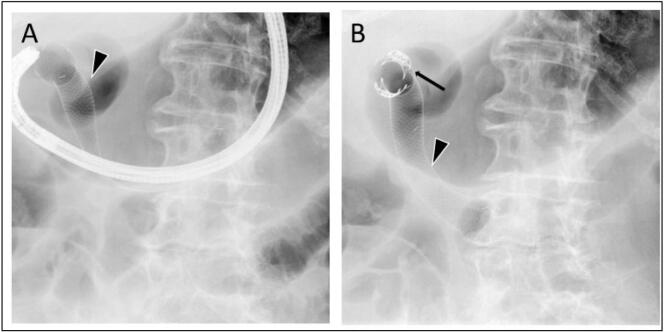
A: Intraoperative X-ray during stent placement by gastroscopy, securing correct insertion and placement of the Niti-S fully covered duodenal stent (black arrowhead) in relation to the fistula. B: X-ray after the procedure was finished, demonstrating the attachment of the stent, secured by two OTSC clips (thin black arrow) in the proximal end of the stent.
